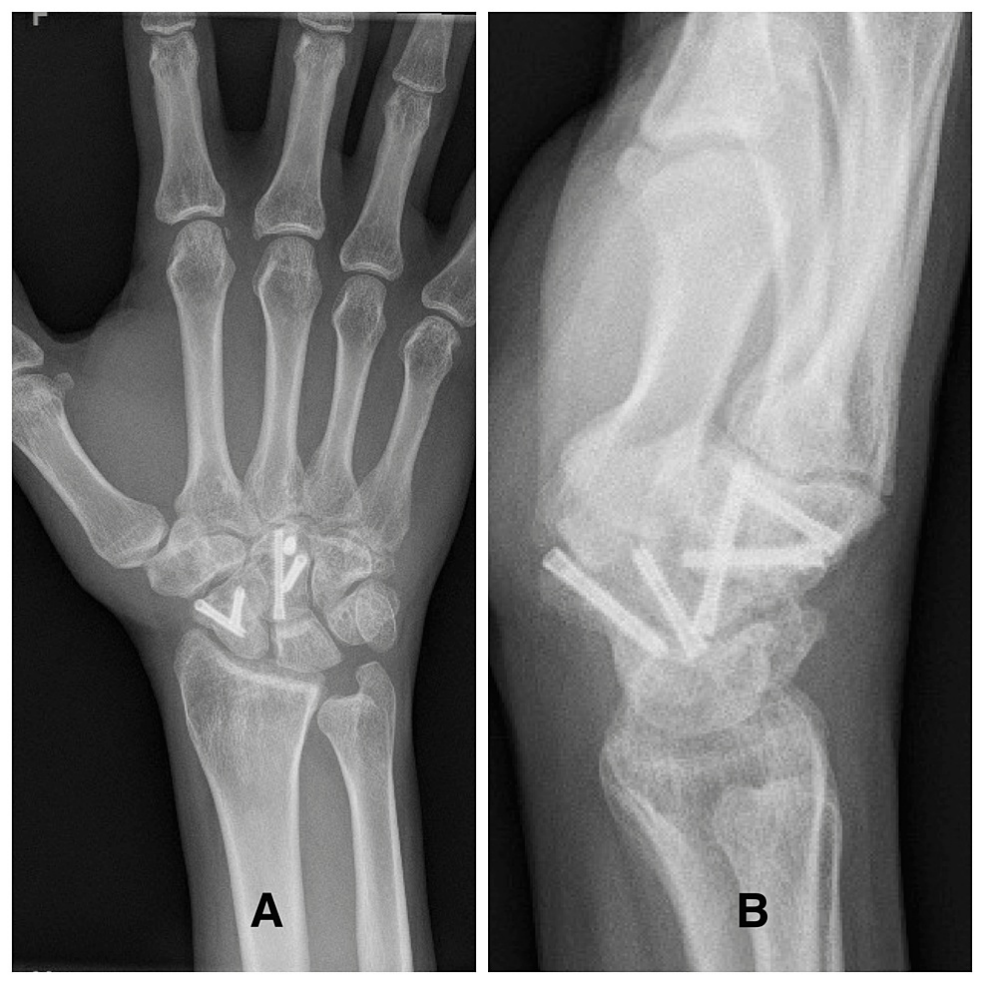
Right wrist X-rays showing open reduction of the fractures and internal fixation at six years postoperatively (A) Anteroposterior view. (B) Lateral view
